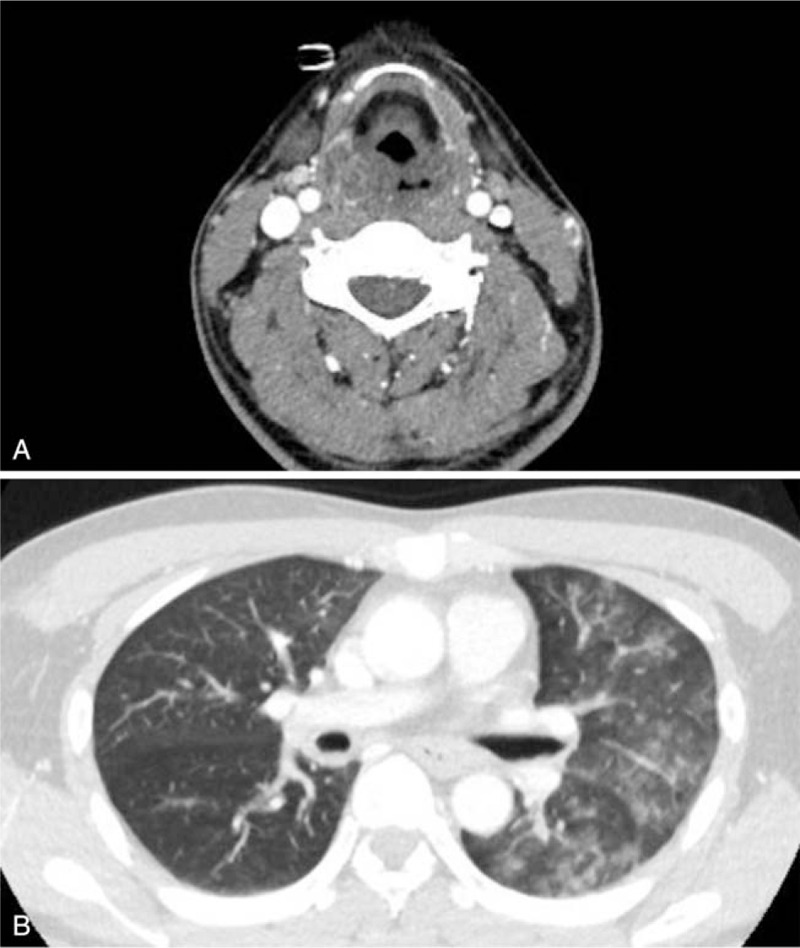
Chest CT findings after acid aspiration on the day of exposure. A. Swelling of hypopharynx and epiglottis is shown. B. Multifocal peribronchial ground-glass opacities shown in the left lung suggest acute lung injury related to acid aspiration.
